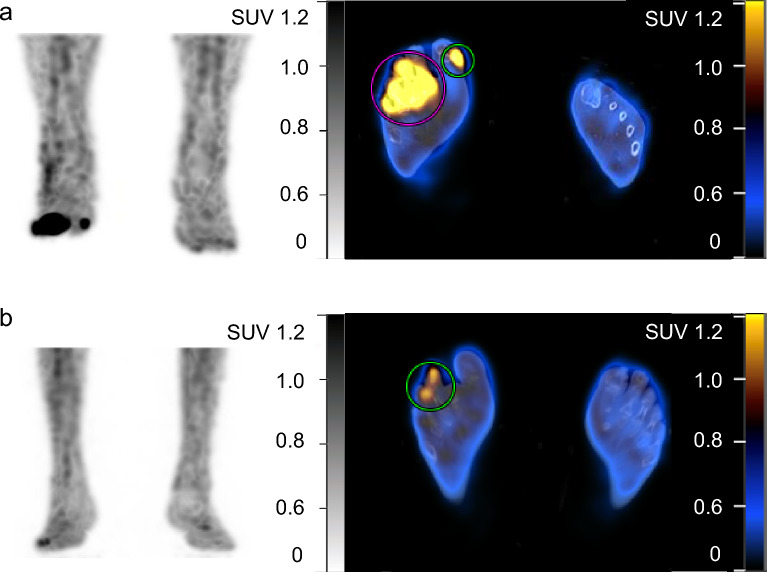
(a) Abnormal leukocyte accumulation was observed in the right 1st to 5th toes, with the radioactive lesions extending to the bone, indicating osteomyelitis (left panel, MIP image; right panel, axial SPECT/CT). Two spherical VOIs were drawn on DFIs and the highest SUVmax was 4.2 (higher than the cutoff of 1.1) Despite intensive treatment, the infection progressed, leading to the patient undergoing right below knee amputation 43 days after SPECT/CT. (b) Mild focal abnormal leukocyte accumulation was detected in the right 3rd and 4th toes. The lesions were in contact with the bone, which raised a suspicion of osteomyelitis (left panel, MIP image; right panel, axial SPECT/CT). A circular VOI was drawn on DFIs and the SUVmax was 1.0 (lower than the cutoff of 1.1). After 10 weeks of antibiotic treatment, the infection was successfully resolved, and the foot was preserved.
